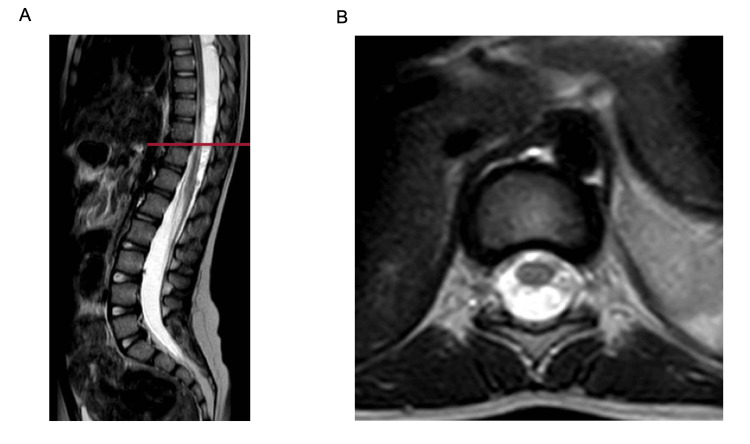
Sagittal (A) and axial (B) T2-weighted image from the initial MRI lumbar spine for preliminary evaluation, showing a dorsal CSF-intensity lesion causing ventral displacement and compression of the thoracic spinal cord.
